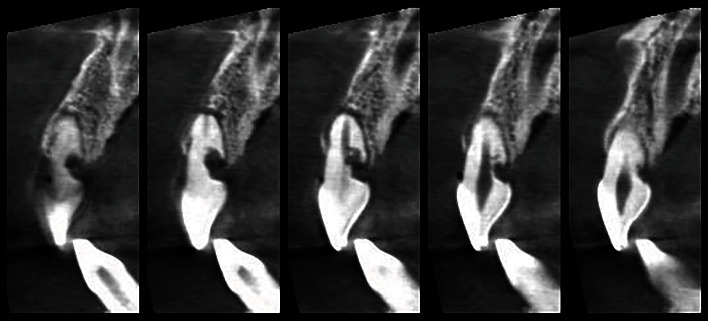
Preoperatory CBCT of the patient.
